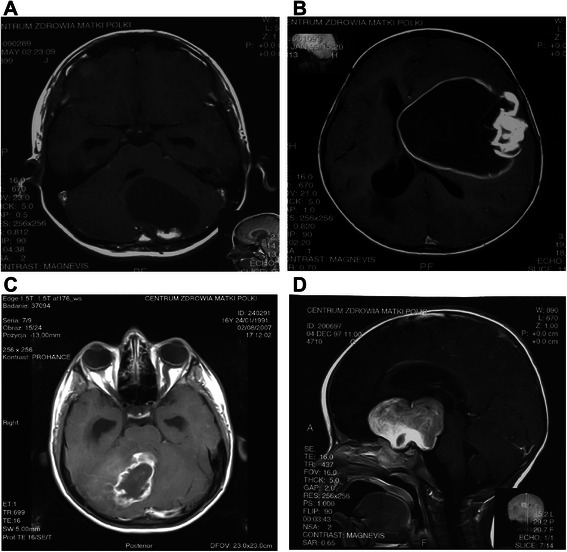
Radiological type of pilocytic astrocytoma. a cystic tumor with an enhancing cyst wall, R1. b cystic tumor with a non-enhancing cyst wall, R2. c solid tumor with central necrosis, R3. d solid or mainly solid tumor, R4. MRI scans after contrast administration
